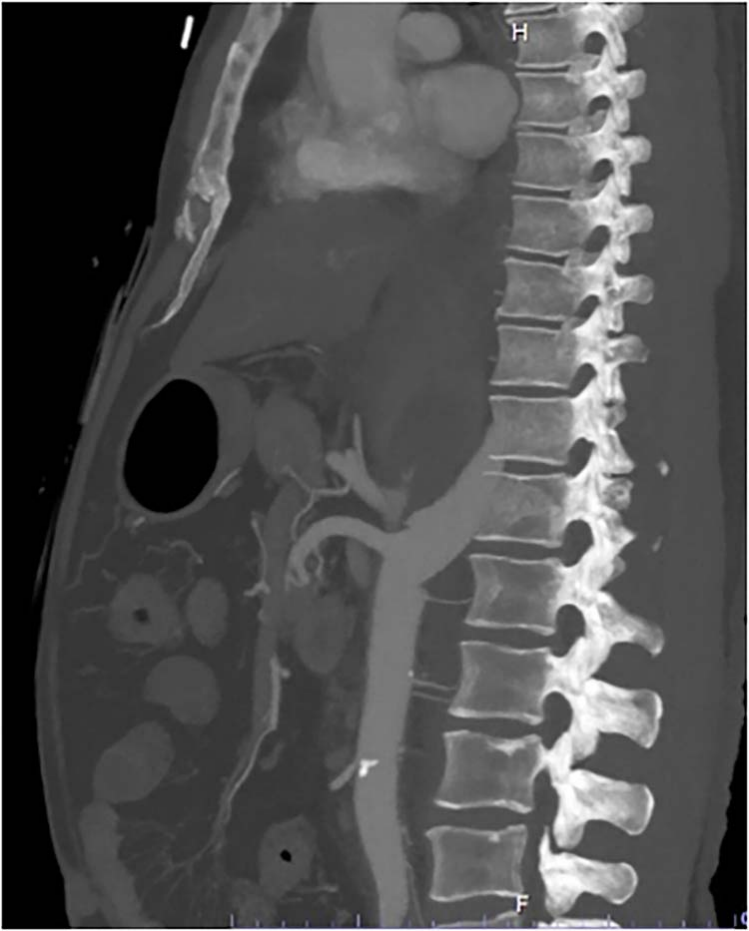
Sagittal CT slice demonstrating coeliac avulsion.
